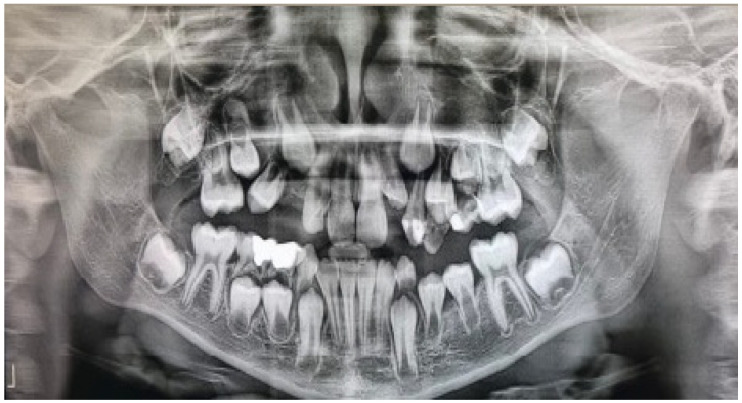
Twelve-month postoperative panoramic radiograph showing the resolution of both lesions.
